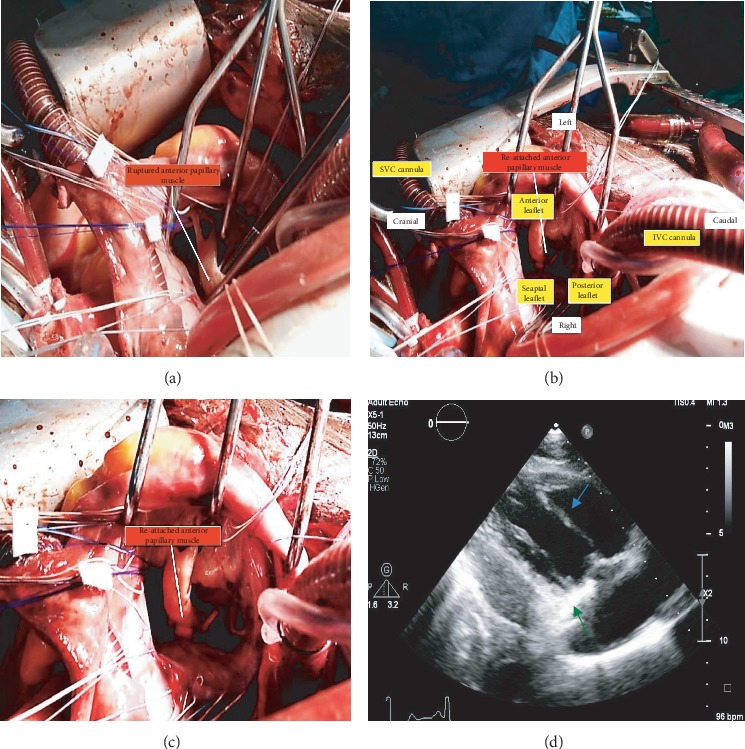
(a–c) Intraoperative images of ruptured anterior papillary muscle and its repair and (d) transthoracic postoperative right ventricular inflow view showing the repaired anterior papillary muscle (blue arrow) and annuloplasty ring (orange arrow).
